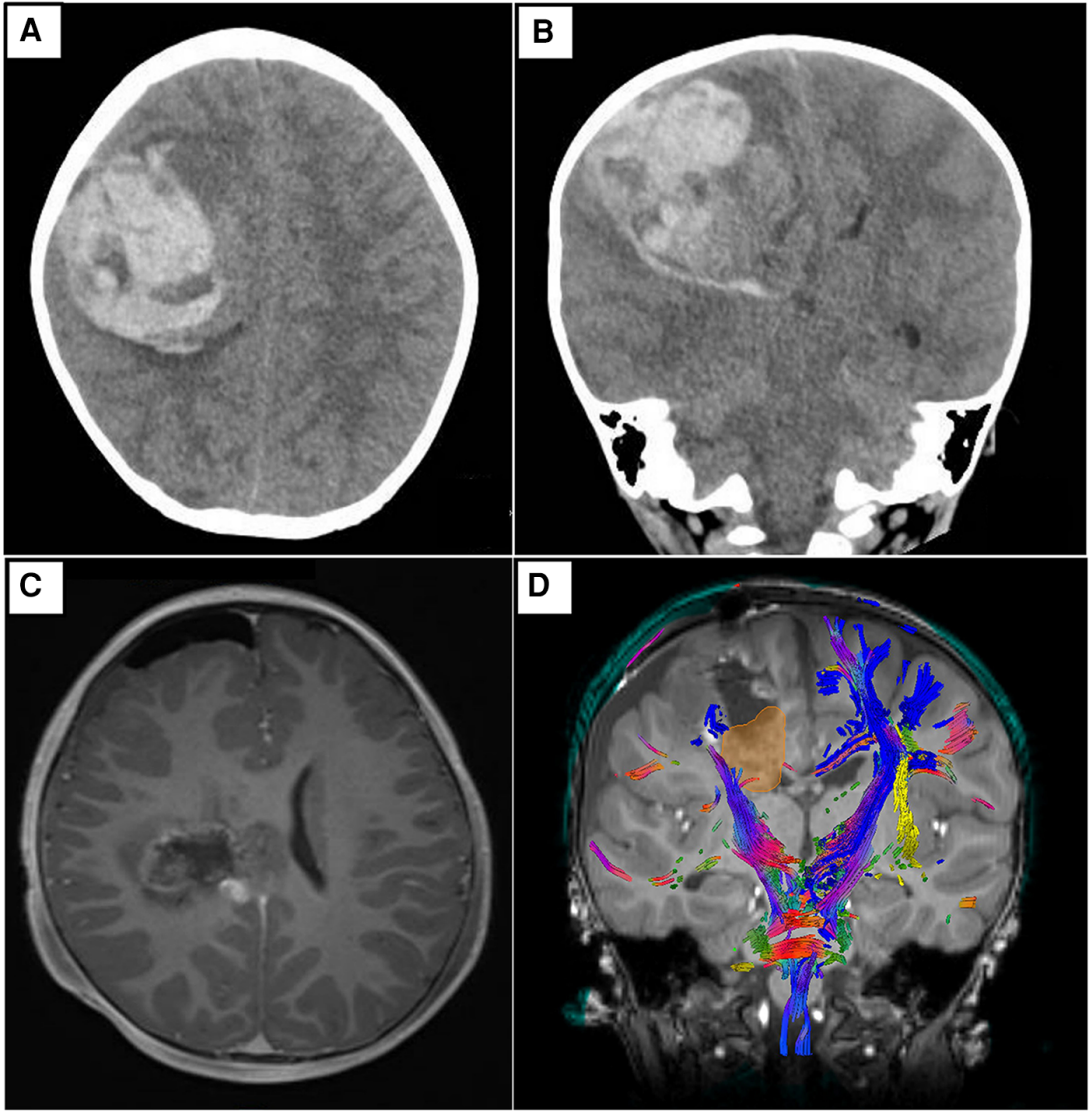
Representative non-contrast CT brain images in axial (A) and coronal (B) views. Both depict a predominantly acute intraparenchymal hematoma (6.8 × 6.4 × 5.6 cm) in the right frontoparietal region, with associated cerebral edema and mass effect. There is intraventricular extension of hemorrhage with early hydrocephalus. Subfalcine and mild right uncal herniation is seen. (C) Representative T1-weighted post-contrast MRI brain images in axial view. There is an irregular, ill-defined lesion with a heterogeneous signal, intermixed with blood products centered in the right frontoparietal region. The previous leftward midline shift has improved. Of note, there are foci of nodular enhancement in the periphery of the lesion that likely represent residual tumor. (D) Representative screenshot of relevant tractography (main corticospinal fibers in blue) in relation to the tumor (yellow-gold) from Modus Plan (Synaptive Medical, Toronto, Canada). This is a neurosurgical planning software that uses preoperative diffusion MRI data to segment out the patient's white matter tracts.
